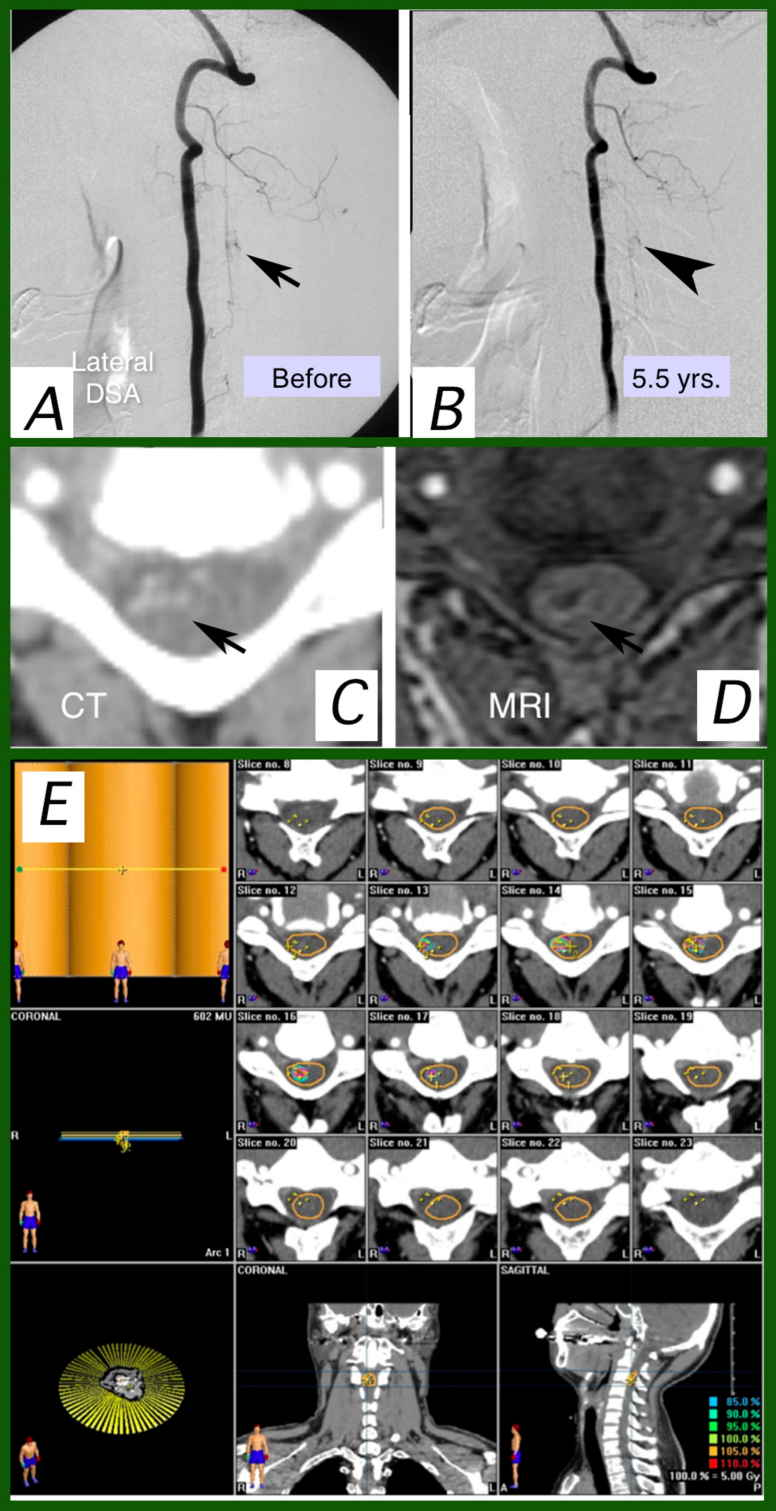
Case 1 1A: Lateral views of right vertebral angiography before and (1B) five and a half years after stereotactic radiotherapy (SRT). 1A: small nidus fed by the anterior spinal artery and draining posteriorly (arrow); 1B: arteriovenous shunt flow disappeared (arrowhead), though the dilated vessel located posteriorly was still observed; 1C: the nidus was located on the right side of the spinal medulla as a contrast-enhanced area (arrow) with vessels on pre-SRT CT; 1D: pre-SRT gadolinium-enhancement spoiled gradient echo MRI was not very useful to delineate the nidus because low-intensity hemosiderin affected the surrounding findings (arrow); 1E: dose-planning of SRT on BrainSCAN workstation. A three-arc cone-collimator plan was employed. CT: computed tomography; MRI: magnetic resonance imaging; SRT: stereotactic radiotherapy
